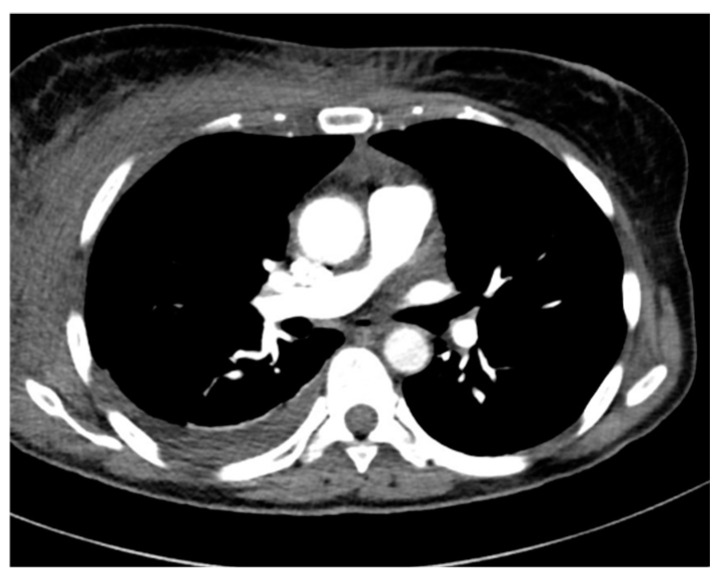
Chest CT. Cellulitis and mediastinitis caused by *S. pyogenes*.
